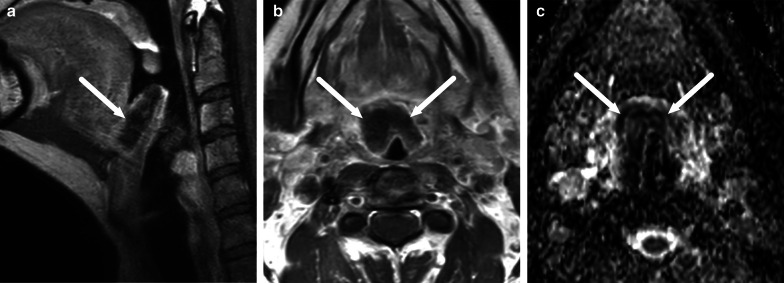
An epiglottic abscess (arrows) on sagittal (a) and axial (b) post-contrast T1-weighted images and on an axial ADC map (c)
